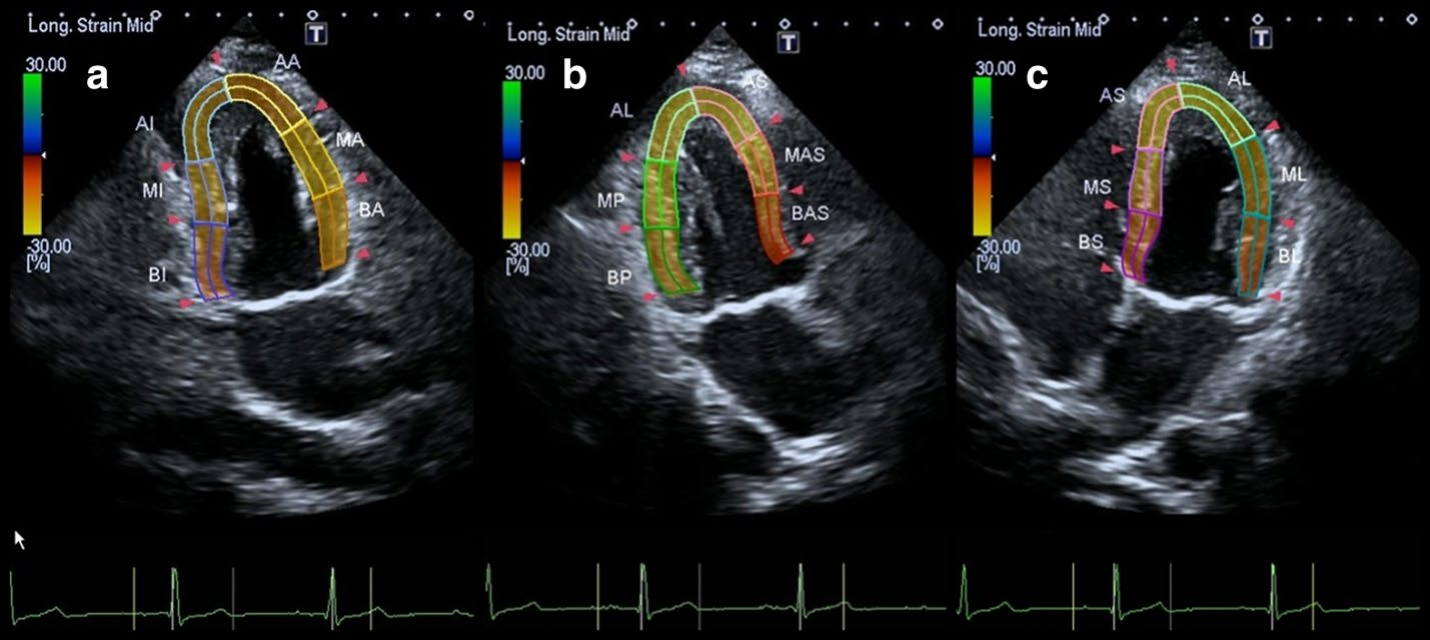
Example of 2DSTE long-axis image. a–c represent the apical 2-, 3- and 4-chamber view, respectively
